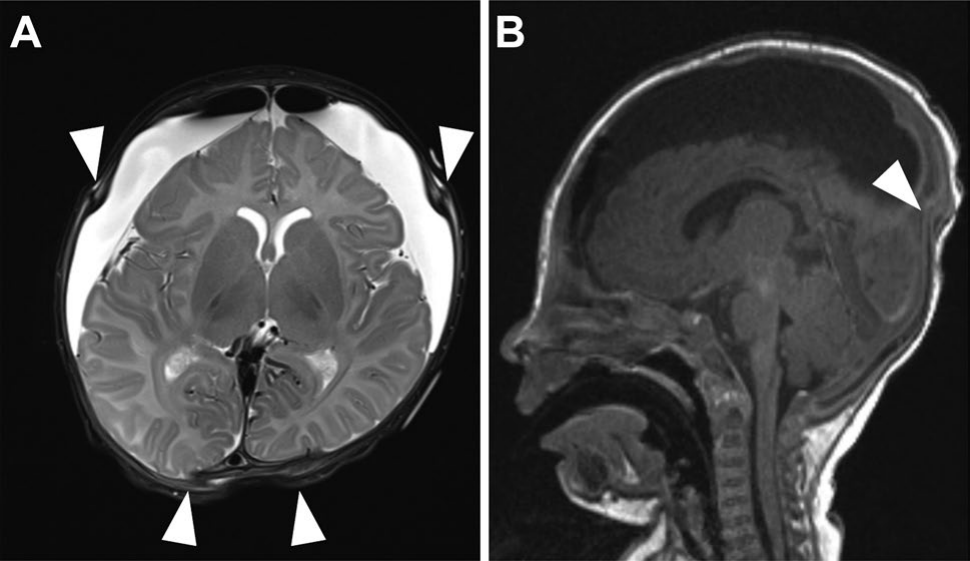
The first postoperative imaging after drainage of the subdural hygroma and insertion of subdural catheter A demonstrates the T2-weighted axial and B the T1-weighted sagittal MRI depicting the slight displacement of the coronal and lambdoid suture after the release of the subdural fluid (arrowheads) indicating significant pressure relieve
